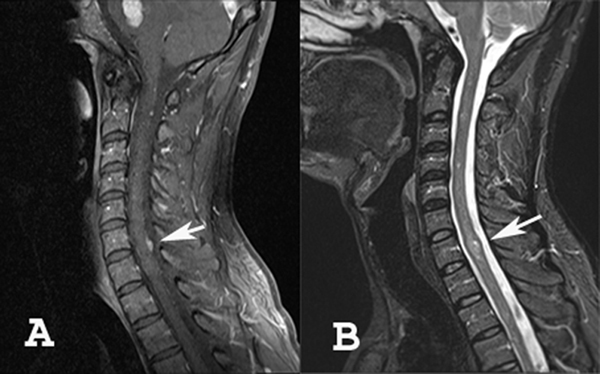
(A) sagittal T1 contrast-enhanced MR image of the cervical spine. A dural-based enhancing mass in seen at the C6-C7 level (arrow), suggestive of meningioma. (B) sagittal T2-weighted MR image of the spine. A focal hyperintense abnormality is demonstrated at the C7 level (arrow), compatible with intramedullary tumor (ependymoma or astrocytoma).
